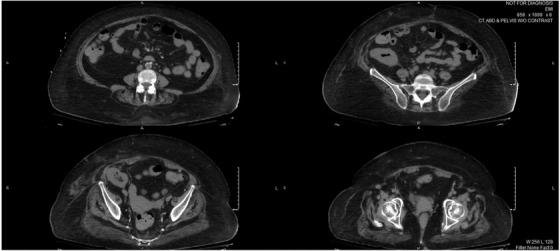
CT images with no contrast showing enlarged para‐aortic lymph nodes.
